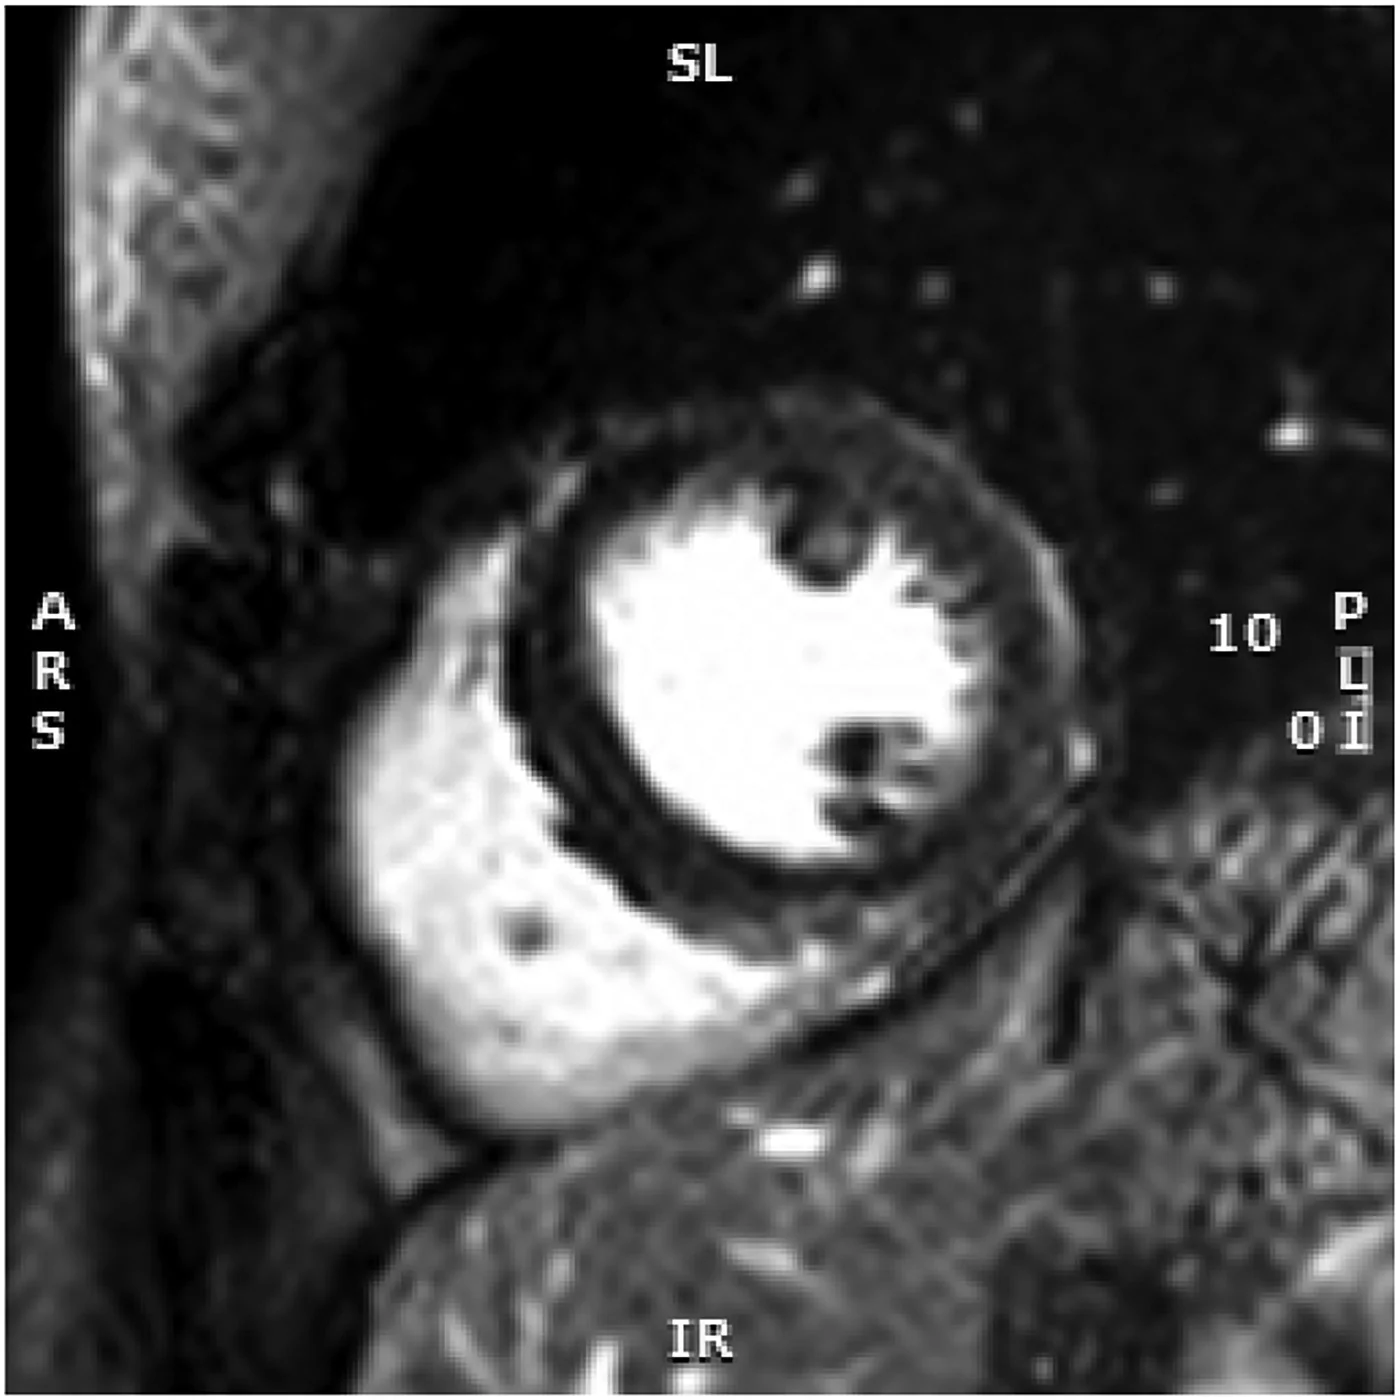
Post-contrast cardiac magnetic resonance image on a mid-ventricular short-axis view demonstrating the absence of late gadolinium enhancement.
